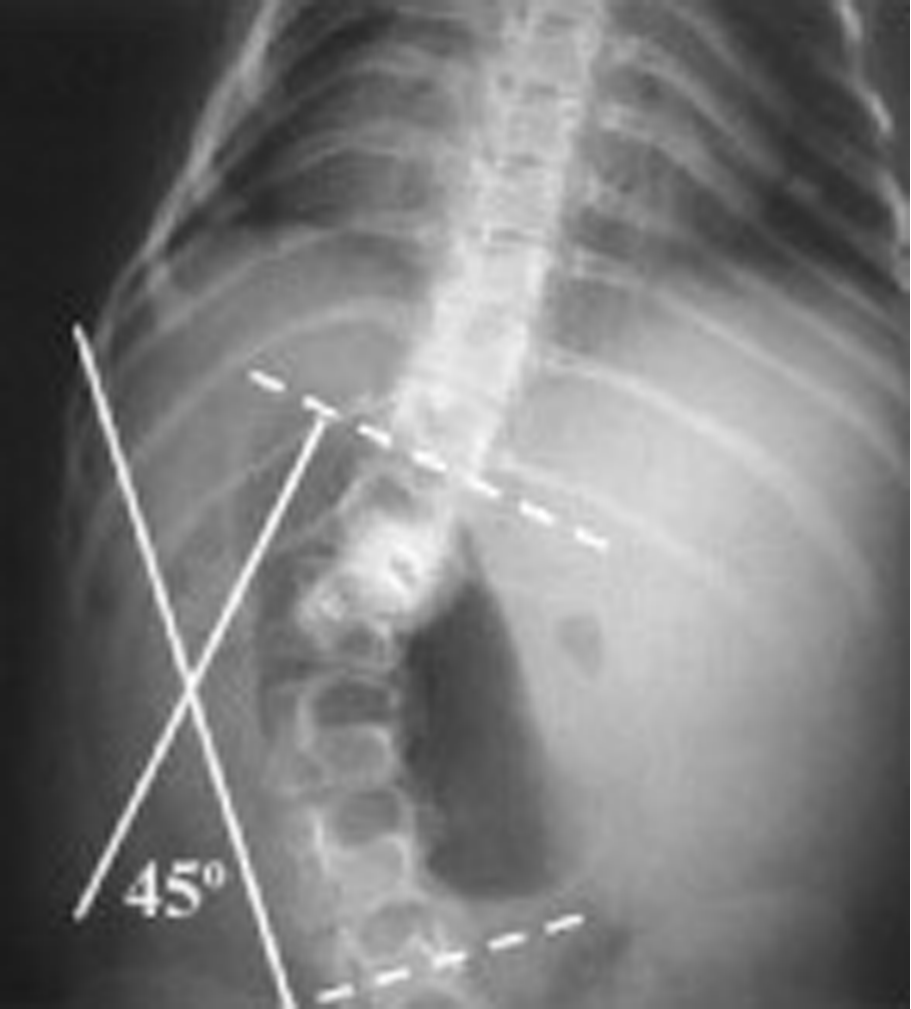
X-ray of lumbar spine demonstrating an apex left 45 degree scoliosis in the same 7 year-old female with MSS [13].
